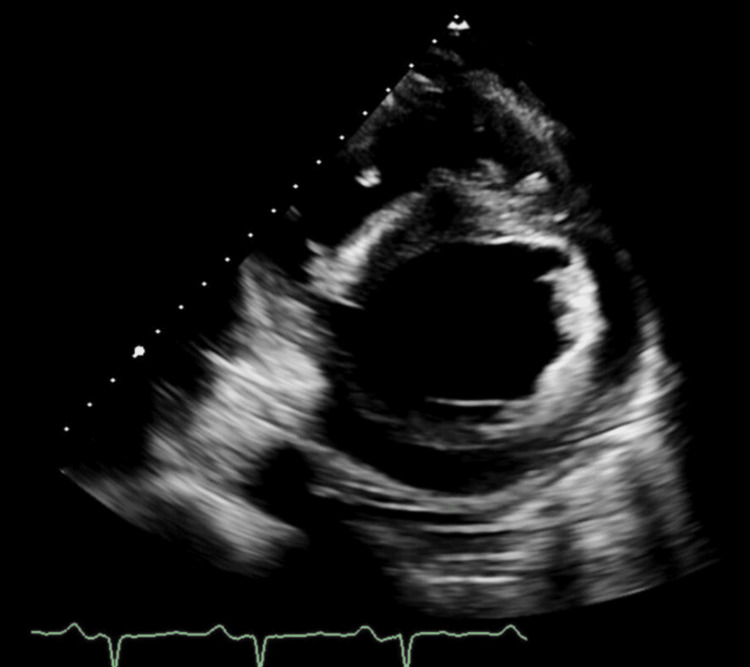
On transthoracic echocardiogram, parasternal short-axis view of the heart in diastole shows concentric left ventricular hypertrophy.
